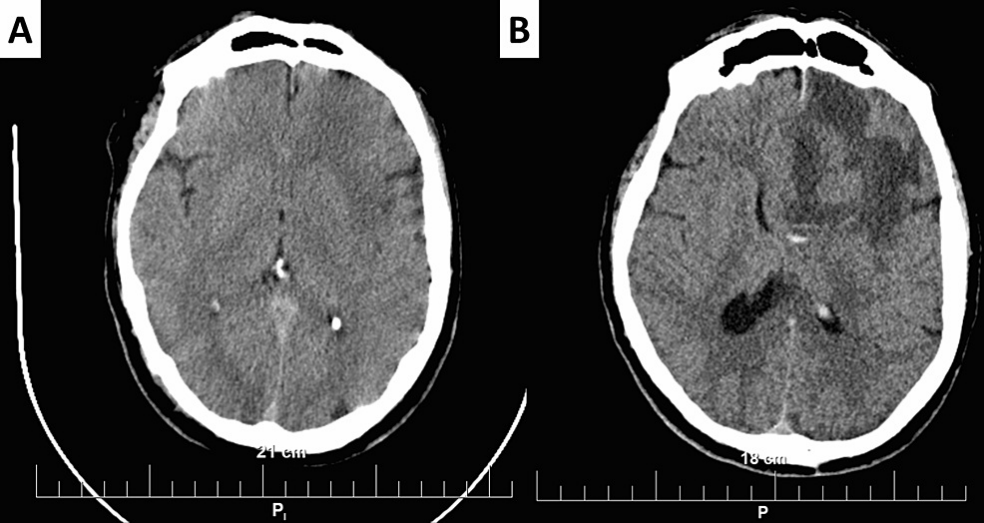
Initial CT (A) and control CT (B) (A) No presence of tumor in the brain parenchyma. (B) Right-sided hypointense tumor with irregular border in the frontal lobe.
